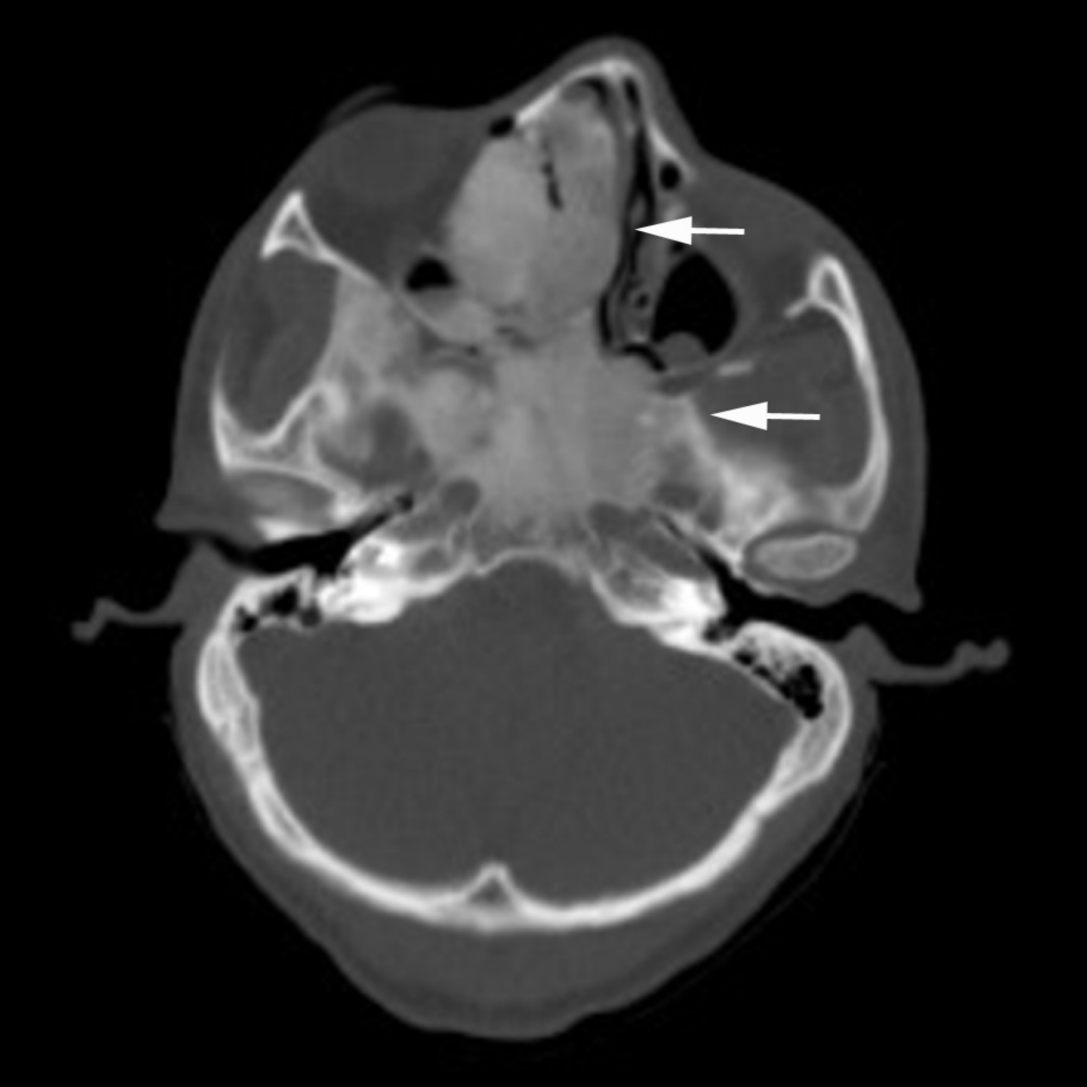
Single photo emission computed tomography (SPECT) head showed typical para-orbital and para-nasal ground-glass lesions as indicated by arrows.
